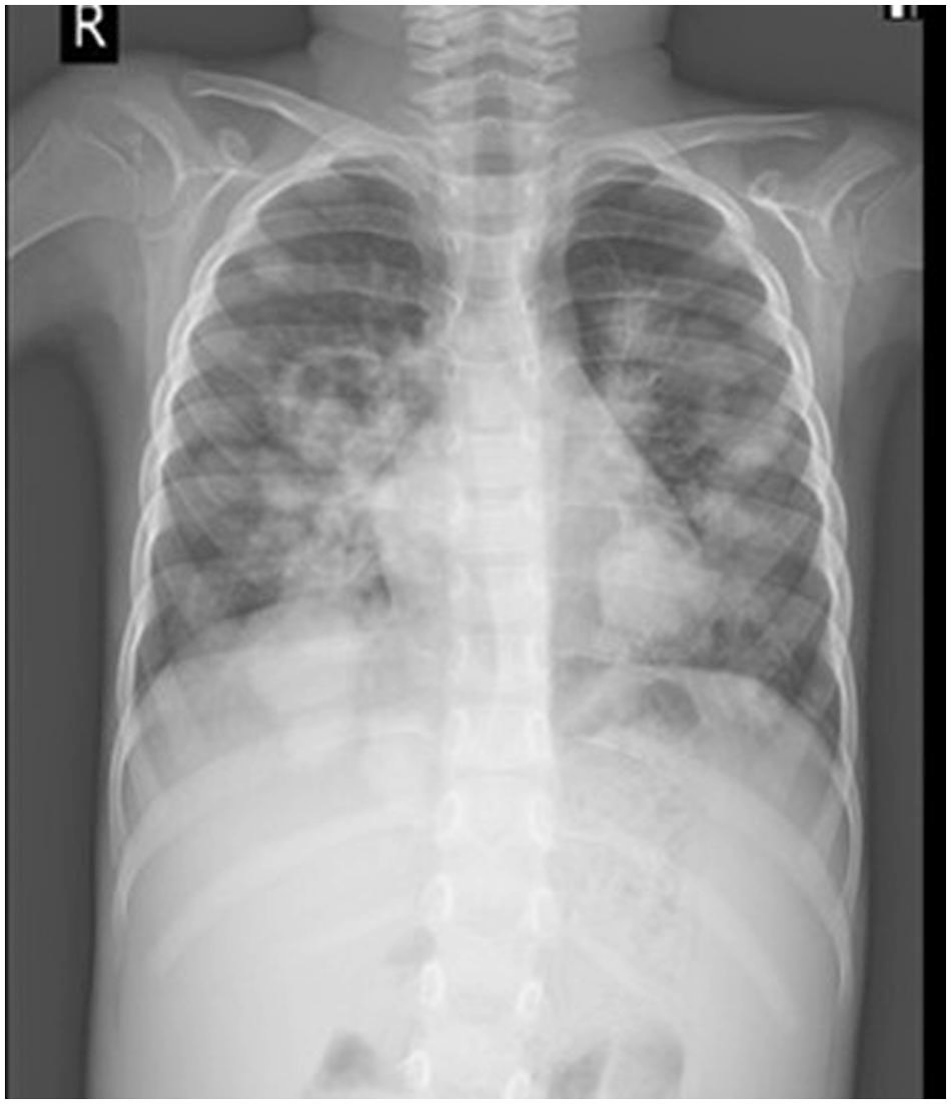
Chest X-ray showing right parahilar opacity with cavitation, opacity in the retrocardiac region, and radio-opacity silhouting the right diaphragm. After this serial, X-rays were done and CT in Figure 2 was done after 1 year due to repeated infections/admissions.
